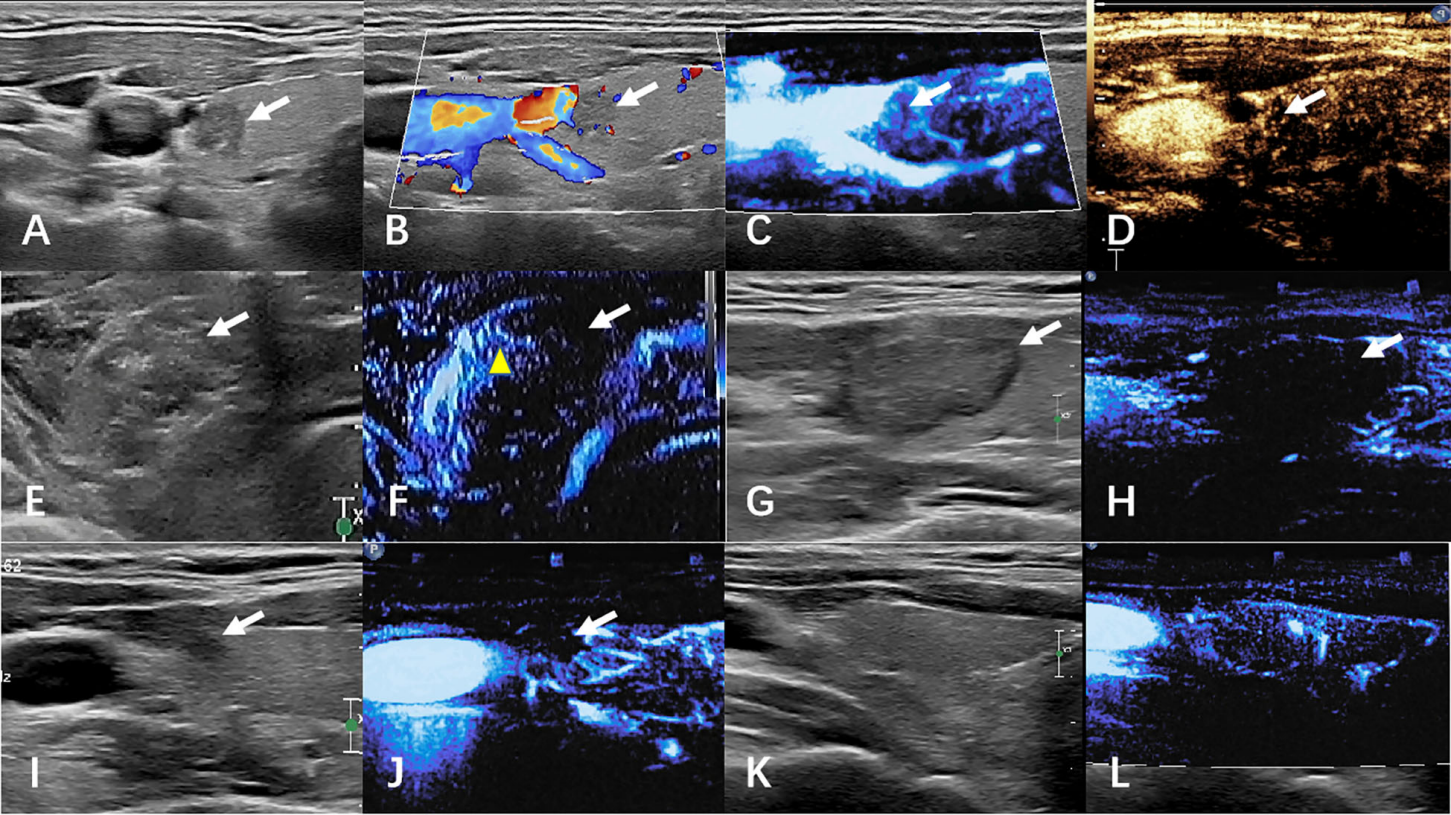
The images of MFI group before and after ablation were compared. (A–D) preoperative ultrasound, CDFI, MFI, and CEUS. (E, F) Ultrasound and CEUS immediately after ablation. (G, H) ultrasound and MFI at 1 month after surgery. (I, J) Ultrasound and MFI at 6 months after surgery. (K, L) Ultrasound and MFI at 12 months after surgery. White arrows indicate lesions. The yellow arrow shows artifacts caused by gas.
